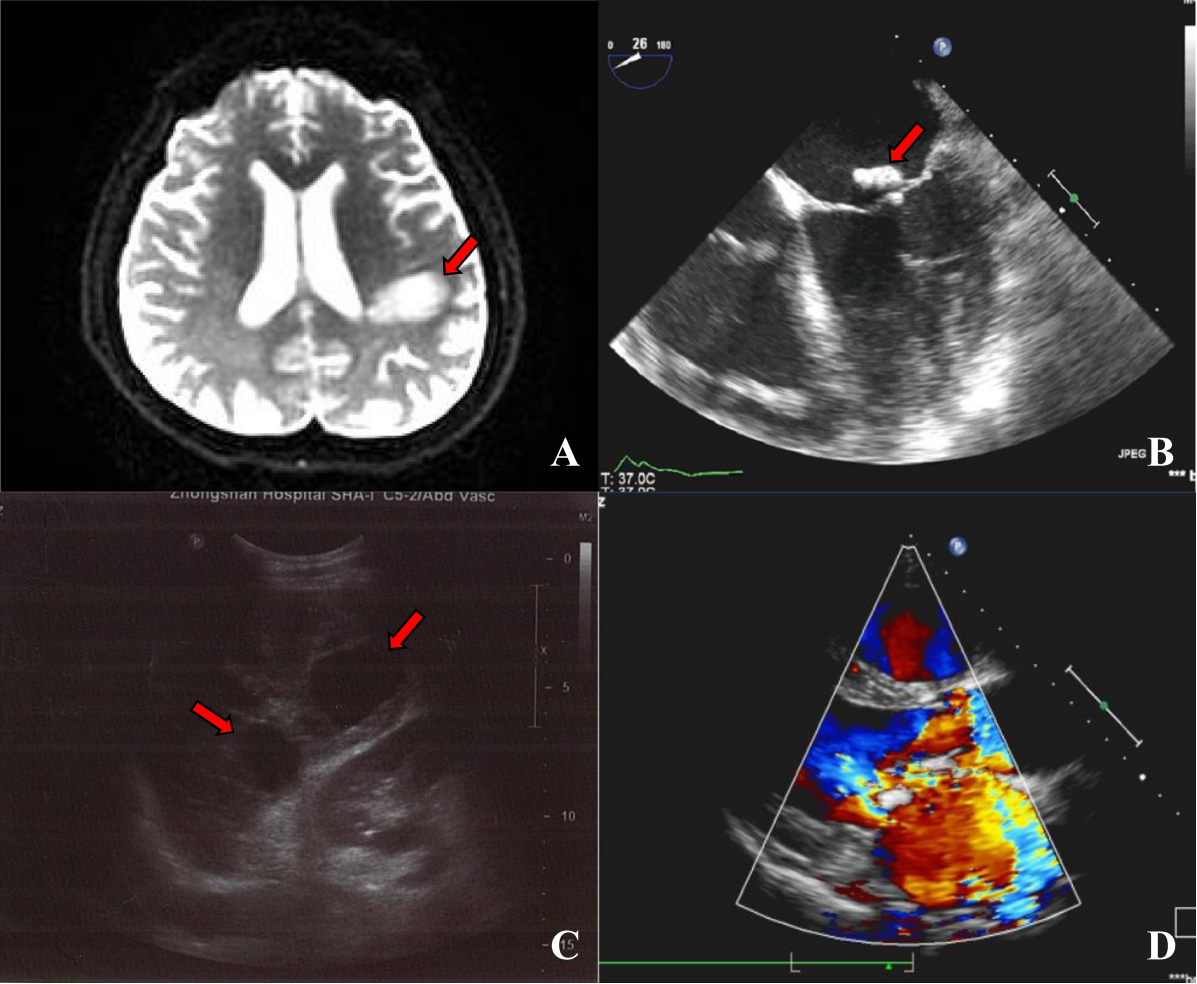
Diagnostic findings. a. Cranial T2-magnetic resonance image showing high signal intensity in the left hemisphere (*red arrow*); b. Echocardiograph clearly shows a sizeable vegetation on the mitral valve (red arrow); c. Abdominal ultrasound showing multiple echo-free areas (*red arrow*) in the spleen, indicating liquefactive necrosis and abscess formation; d. Color Doppler showing severe mitral regurgitation
